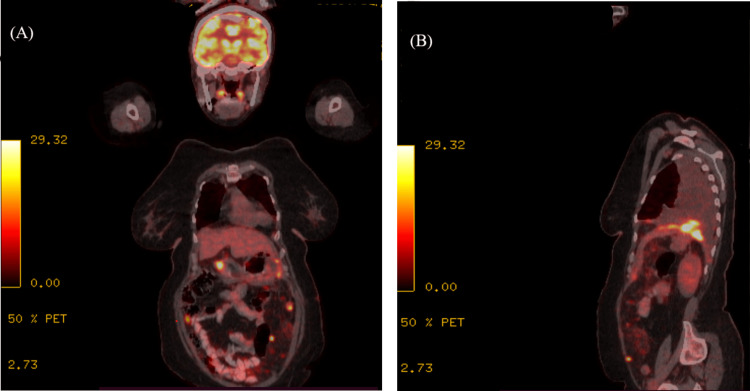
Representative imaging during treatment course. Initial staging PET/CT (Day 20-21) showing multiple metabolically active lesions in the peritoneum, pleura, and liver, in coronal (A) and sagittal (B) projections.
